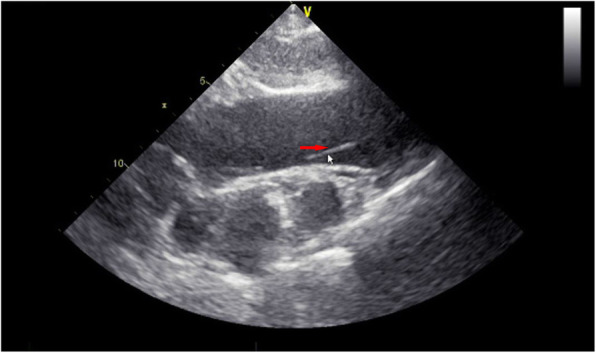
Echocardiography revealed foreign body with strong echogenicity in the ascending aorta and aortic arch (red arrow)
